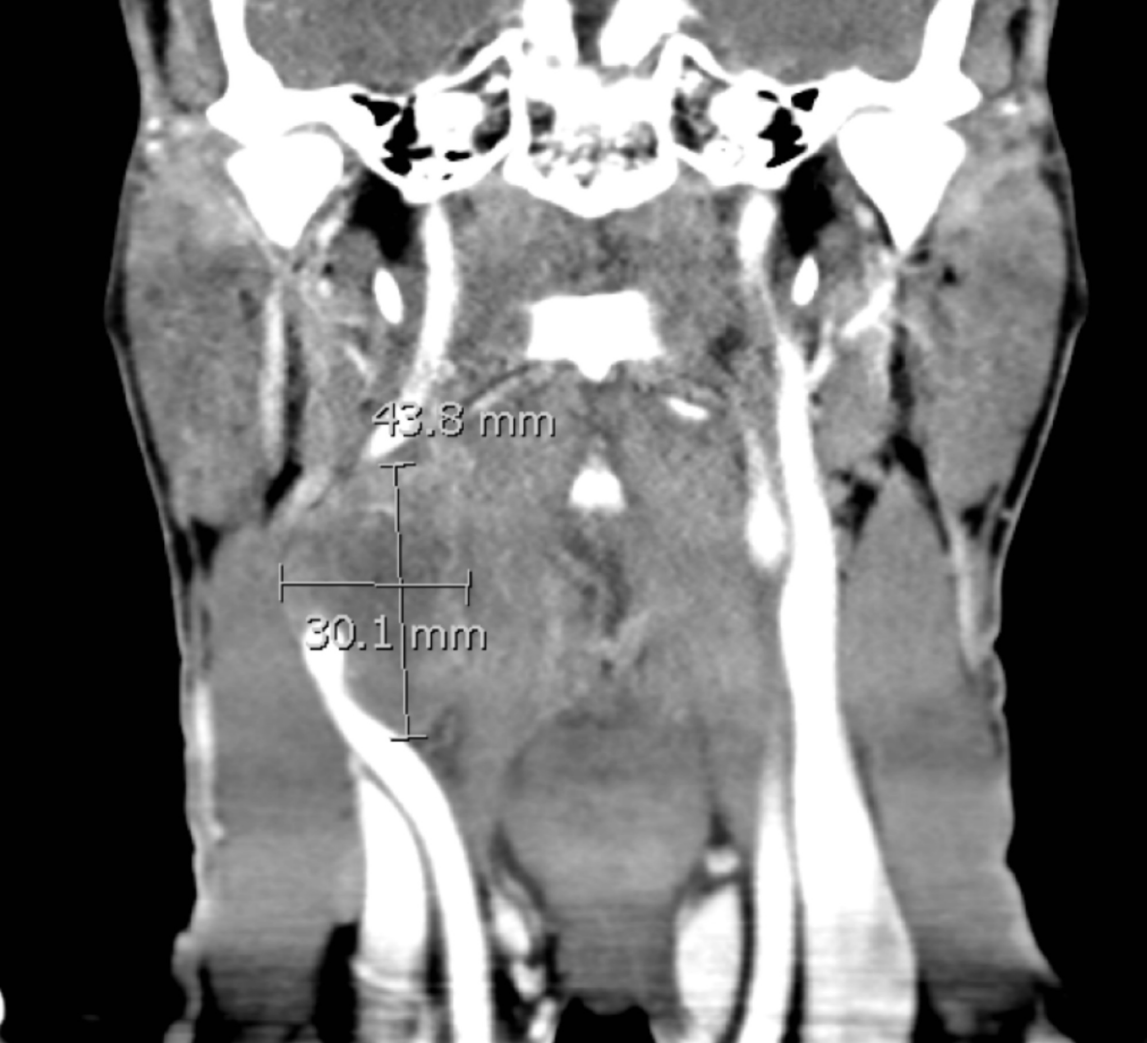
A coronal slice of a contrasted computed tomography scan of the neck, demonstrating a 43.8 mm by 30.1 mm mass at the level of the right common carotid artery bifurcation.
